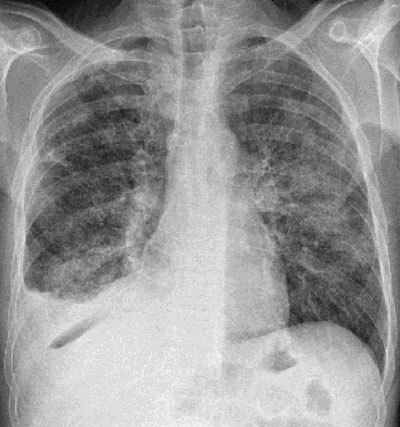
Chest X-ray showing parenchymatous condensation in the middle lobula and a right pleural effusion. A small unusual aeric image is shown under the right section of the diaphragm.
